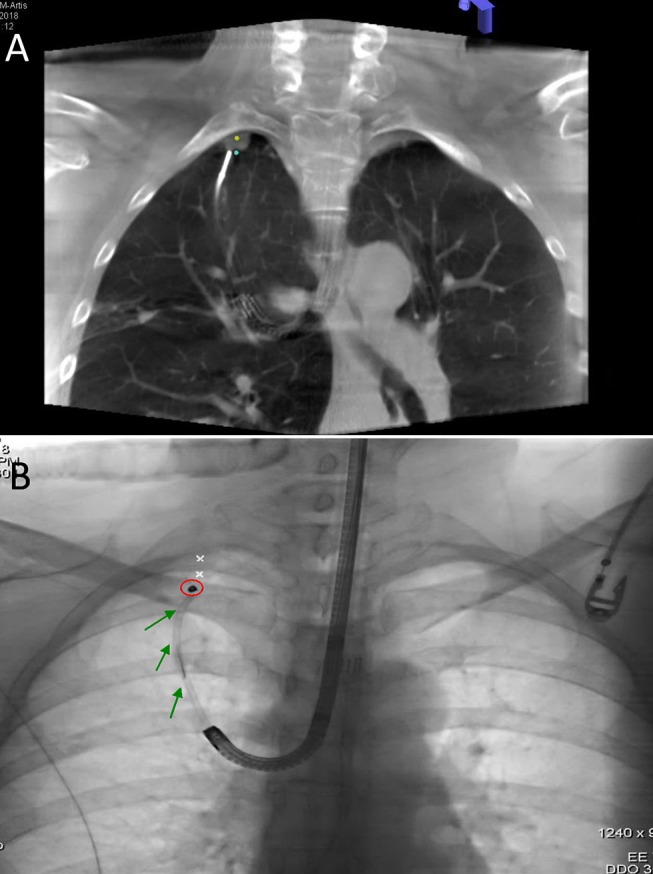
(A) An intraprocedural CT scan confirmed that LG was properly positioned at the deep margin (blue dot) of the nodule of interest (yellow dot). (B) Fluoroscopic view of microcoil (red circle) insertion through EWC (green arrow).
